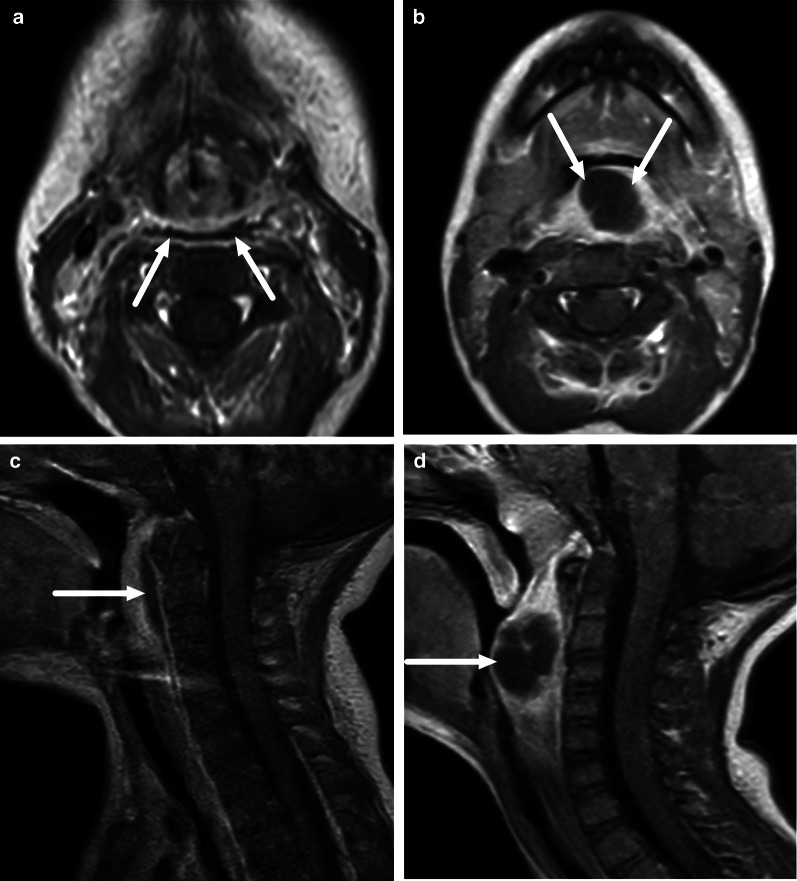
Retropharyngeal abscesses (arrows) on axial (a, b) and sagittal (c, d) post-contrast T1-weighted images in two children (a and c, b and d). The first (a, c) represent a true retropharyngeal abscess as a thin collection between fascial planes, whereas the second (b, d) is a focal collection due to suppurative lymphadenitis. Image modified from ref. [8]
